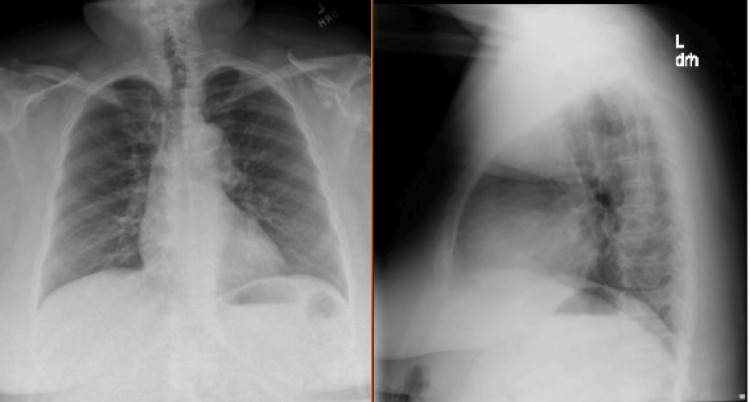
Chest X-ray (posteroanterior and lateral views) Chest X-rays include a posteroanterior (PA) view on the left and a lateral view on the right. The radiographs show clear lung fields without focal consolidation, interstitial infiltrates, cavitary lesions, or nodularity, with no evidence of opportunistic pulmonary infection.
